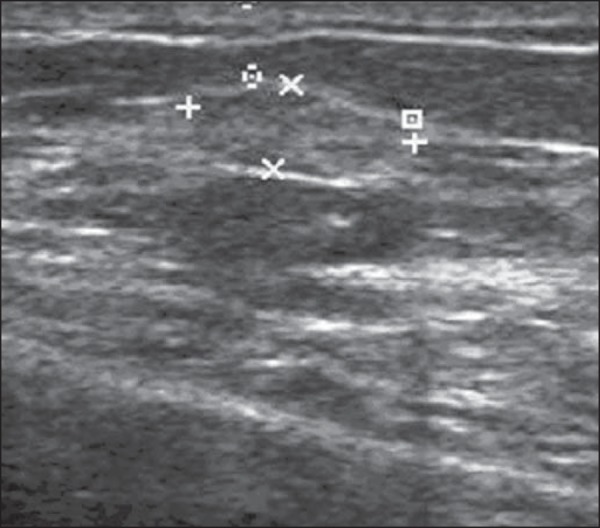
Lymphoma*. Ultrasonography showing a slightly
